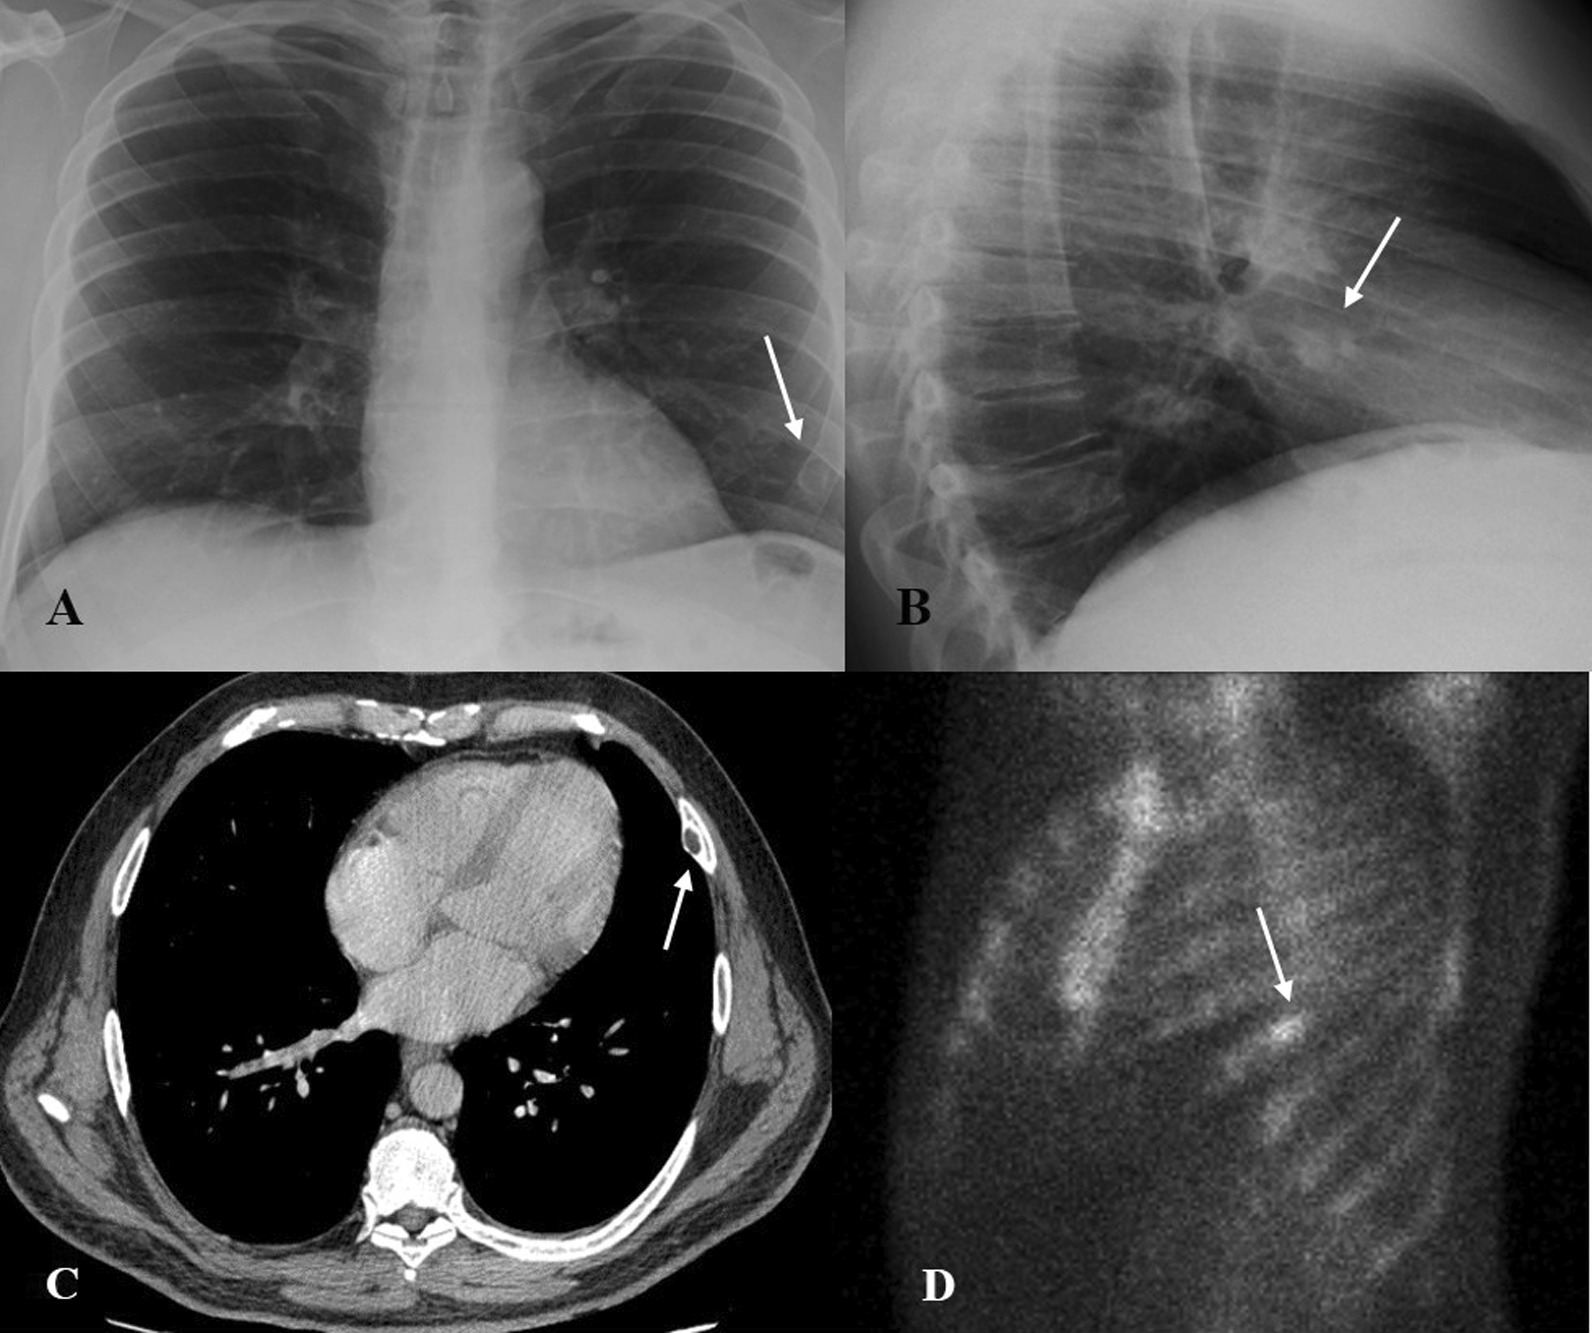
Preoperative imaging: A Chest X-ray shows an indefinite opacity in the left anterior arch of the 6th rib (white arrows), postero-anterior projection; B Latero-lateral projection of Chest X-ray; C CT scan showing a centimeter area with a central swelling and a surrounding sclerosis (white arrow) D An isolated hyperfixation of 6th rib is detected on 99m-tc HDP bone scan (white arrow)
